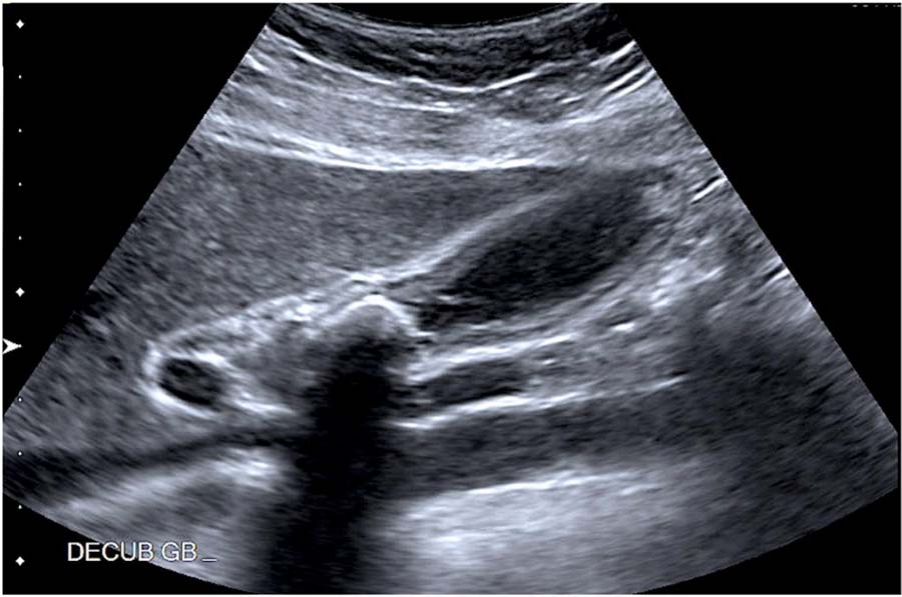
Abdominal ultrasound showing a 2.1 cm calcified gallstone in the gallbladder neck, and a thickened wall.
